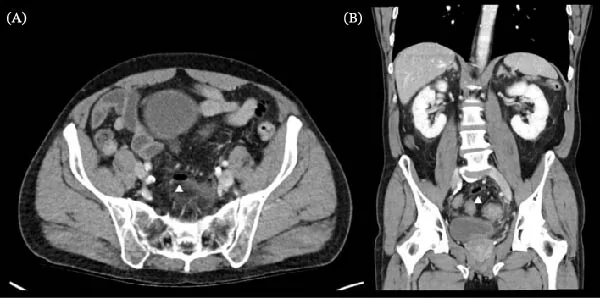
Admission computed tomography scan of the abdomen and pelvis in the portal‐venous phase demonstrating coronal (A) and axial (B) views of sigmoid diverticulitis with findings of retroperitoneal free gas and pelvic fluid collection (white arrows) consistent with a localised perforation.
